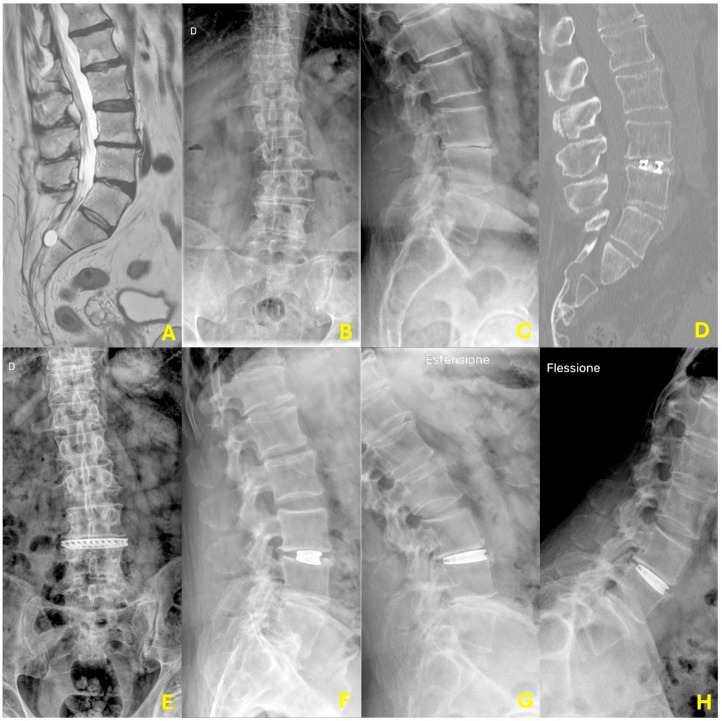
Case presentation. This is the case of a 66-year-old gentleman, suffering from Pfirrman grade IV degenerative disc disease with Modic change at L3-L4 (A), significantly reduced disc height and segmental lordosis (B,C), and 3 mm lateral listhesis of L3 on L4 on the convexity side. The postoperative CT scan (D) and X-rays (E,F) show the implanted 3D printed porous titanium cage correctly positioned at L3-L4, restoring the disc height and the segmental lordosis without subsidence in the inferior endplate. In the one-year dynamic x-rays (G,H), there is no evidence of residual segmental movement or subsidence, while the segmental alignment and disc height are maintained.
